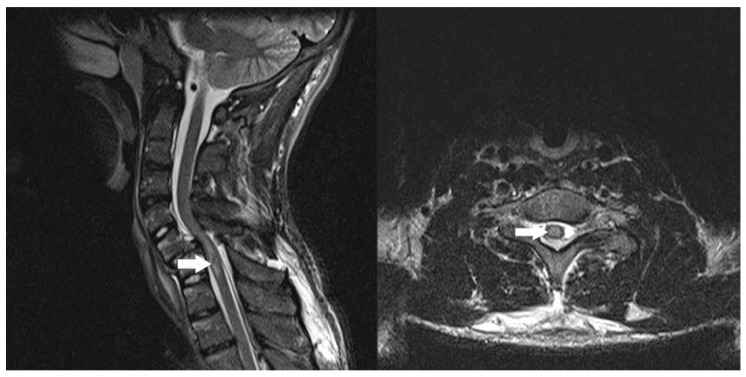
In spinal cord Magnetic Resonance Imaging (MRI) of patients who suffered traumatic spinal cord injury (TSCI), spinal cord hemorrhage and edema were characterized by increased T2-weighted signal within 72 h of injury. This non-contrast T2 weighted, short tau inversion recovery (STIR) sequence MRI depicts the sagittal and axial cross sections of a 17-year-old male 8 h after suffering from a ski accident. White arrows which depict increased T2 signal within the spinal cord indicate edema consistent with traumatic spinal cord injury (TSCI).
